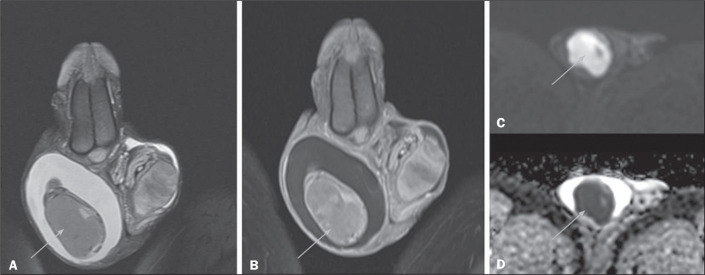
Testicular lymphoma. Coronal T2-weighted image with fat suppression
